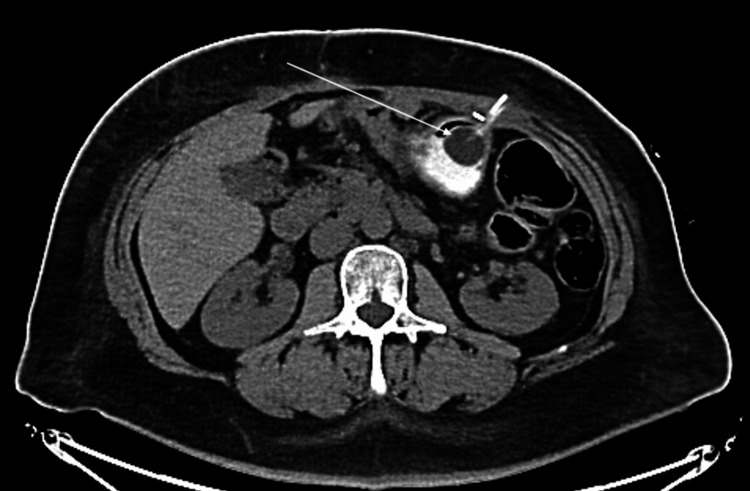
Follow-up CT confirming intraluminal gastrostomy tube position Follow-up CT image demonstrating gastrostomy tube position (white arrow) within the excluded stomach with contrast confirmation of intraluminal placement.
